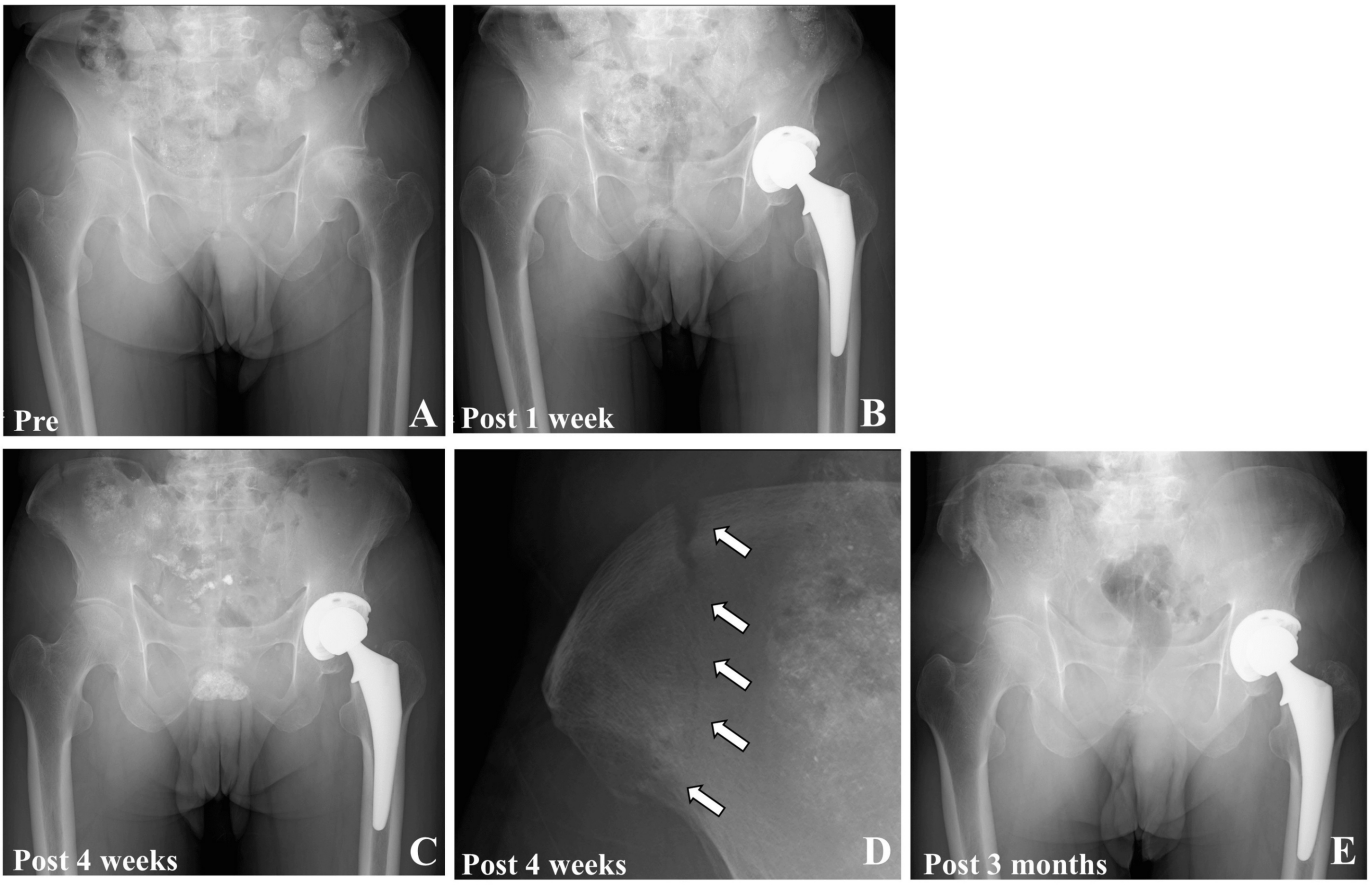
Pre and postoperative X-rays of case one A: Preoperative pelvic X-ray diagnosed as left femoral head osteonecrosis; B: Postoperative X-ray one week after surgery; C: X-ray at four weeks postoperatively showing stress fracture of the right iliac crest; D: Enlarged view of the right ilium from panel C. The white arrow indicates the fracture line of the iliac crest. E: Postoperative X-ray after three months showing slight displacement and bone union of the iliac crest fracture.
